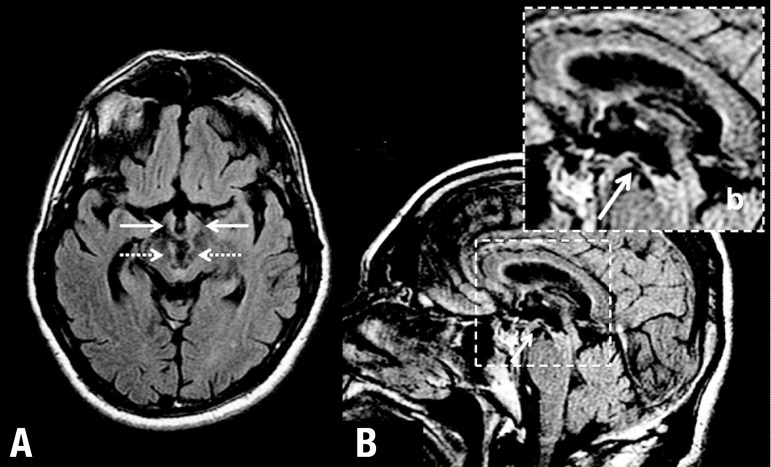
MR - FLAIR acquisition. [A] (axial section): solid arrows
